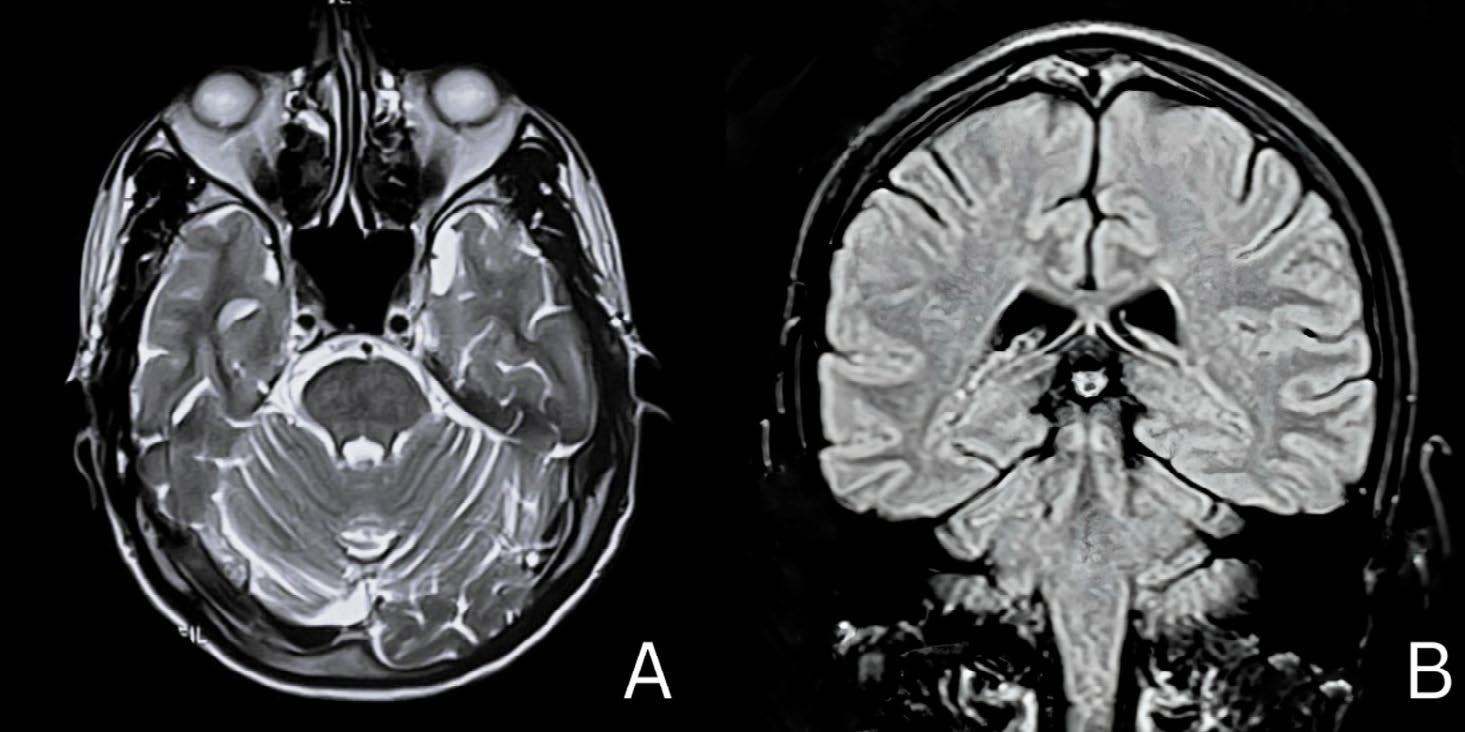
A follow‐up magnetic resonance imaging (MRI) of the brain, (A) T2‐weighted axial image and (B) T2‐weighted FLAIR coronal image showing no evidence of residual disease.
